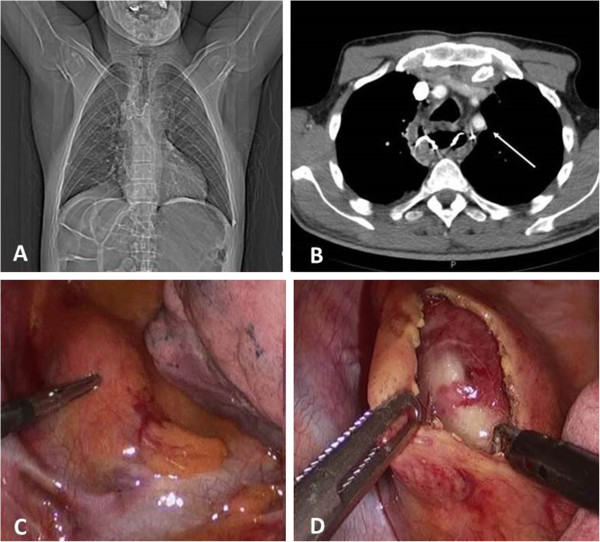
Appearance of the dental prosthesis at CT scan (A-B), and thoracoscopic exposure of the upper thoracic esophagus (C-D).
